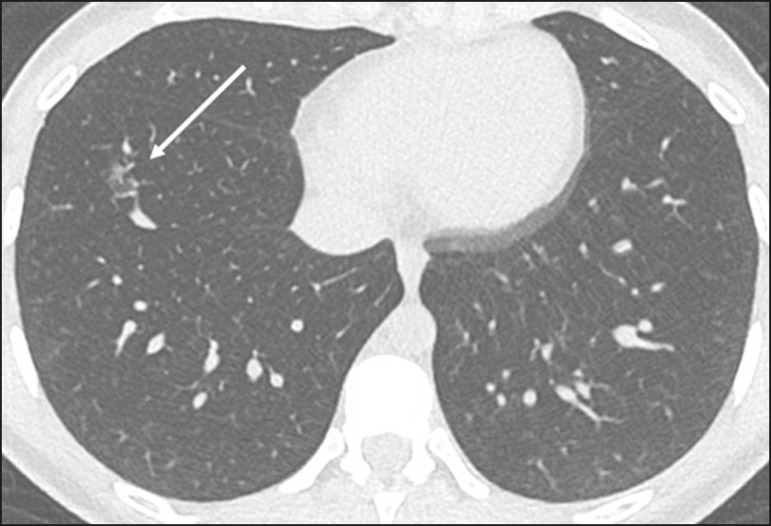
Undetermined viral pneumonia pattern on high-resolution CT of the chest, with tenuous unilateral ground-glass opacity (arrow).Atypical viral pneumonia pattern (Figure 12)-absence of typical or indeterminate findings and presence of lobar/segmental consolidation (without ground-glass opacities), septal thickening with pleural effusion, centrilobular micronodules, or cavitiesFigure 12 f12:
